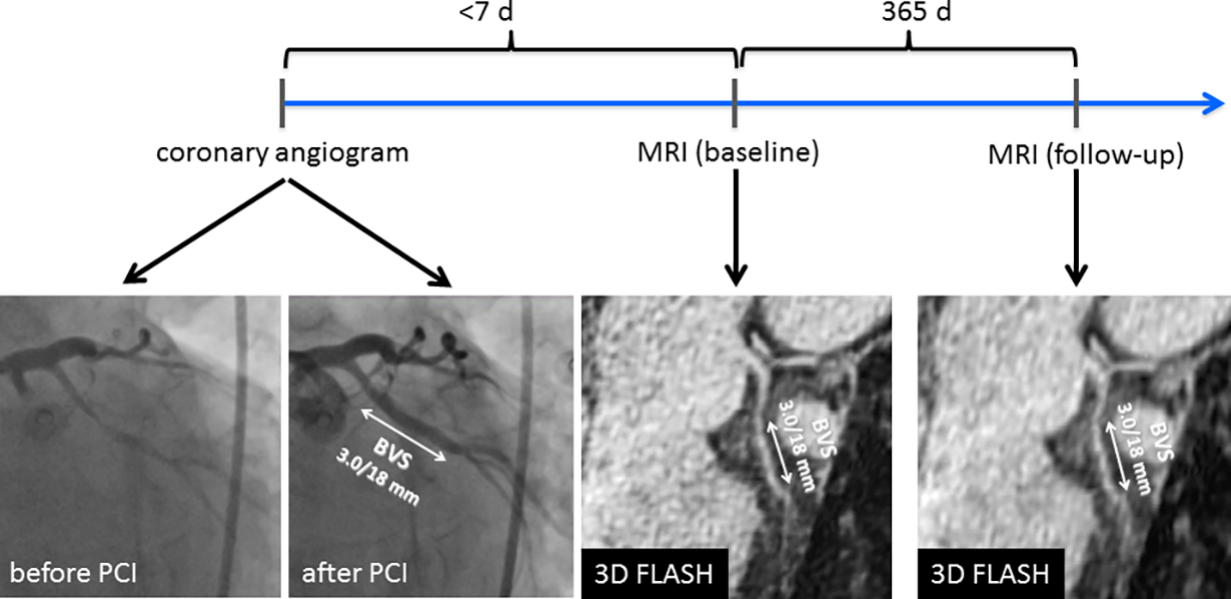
BVS-implantation in the proximal left circumflex artery (LCX). Left: coronary angiogram after implantation of a 3.0/18mm BVS. Middle: MRI at baseline after 2 days, showing the patent scaffolded segment in bright blood (= 3D FLASH) sequences. Follow up one year later confirms the patent vessel in 3D FLASH.
